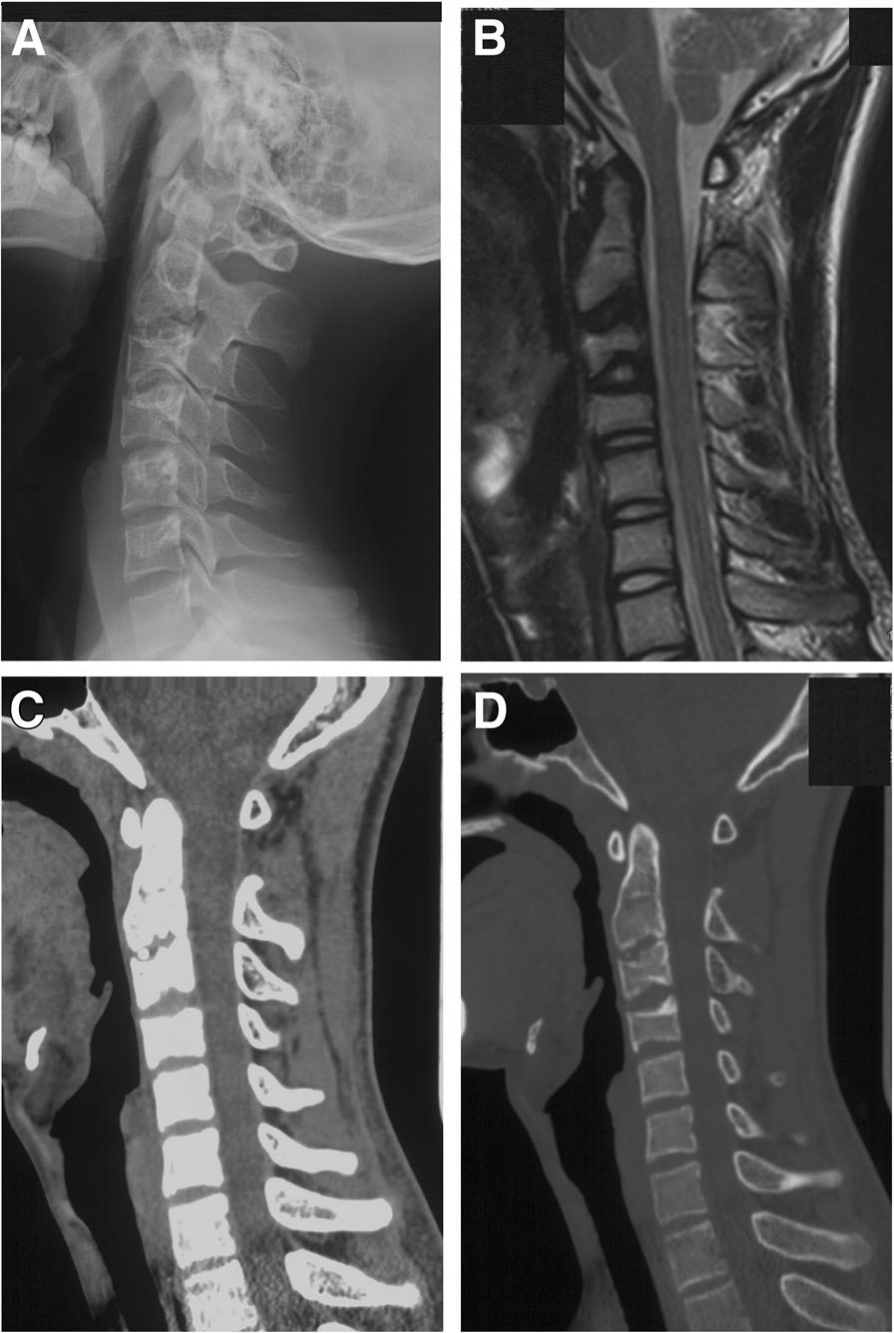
Radiological imaging at 9-year follow-up. a Lateral cervical spine radiograph showed minor IDC remained at C2/3. b Magnetic resonance imaging revealed loss of T2-weighted signal intensity of C2/3 disc and decrease of T2-weighted signal intensity of C3/4 disc. c and d Computed tomography revealed minor calcification at C2/3 intervertebral disc left, IDC and OPLL at the C3/4 level disappeared. Narrowing of C2/3 intervertebral space, flatting of C3 body, widening of posterior edge of C3/4 disc were observed
